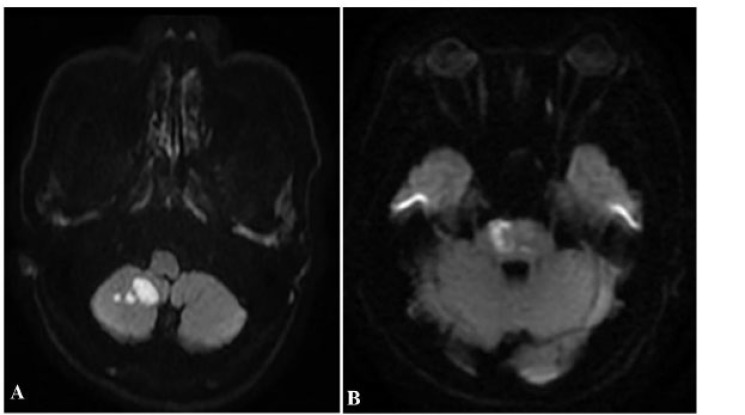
Brain MRI. Acute infarction in the (A) right cerebellar hemisphere and (B) right pons.
